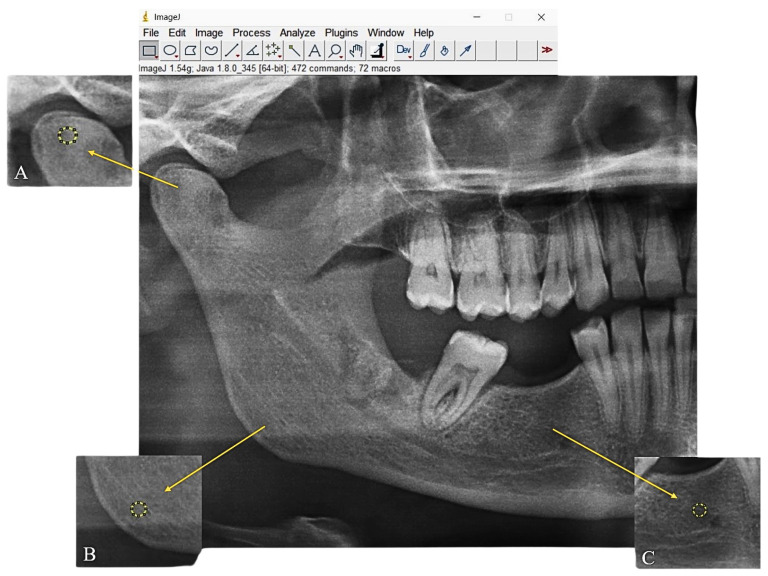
Panoramic radiograph displaying the selected regions of interest (ROIs). (A) ROI-1: mandibular condyle, (B) ROI-2: center of mandibular angle, and (C) ROI-3: mandibular molar area.
